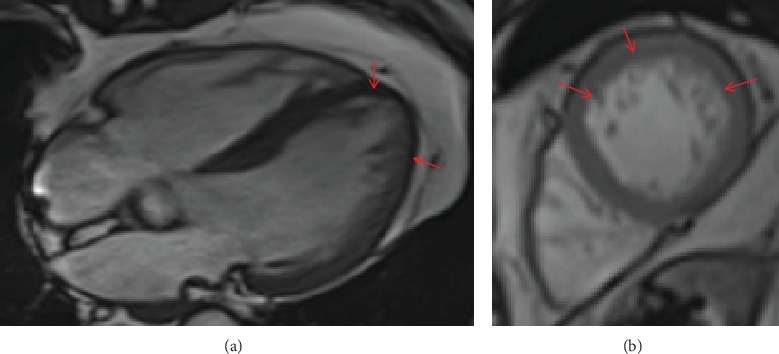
Cardiac MRI (3T, true fisp) showing left ventricular hypertrabeculation/noncompaction in the end-diastolic 4-chamber view (a) and in the apical short axis view (b).
